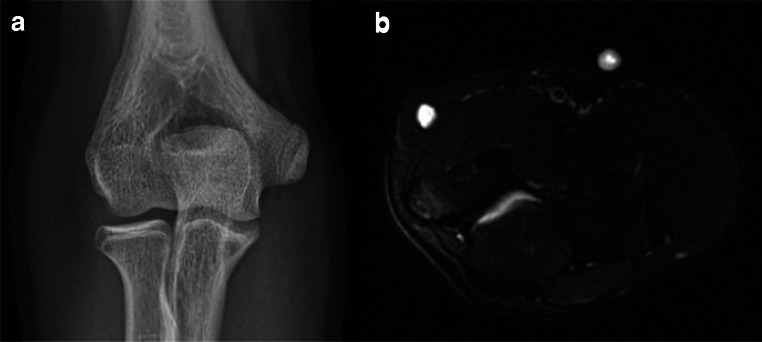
a Anteroposterior radiograph of the elbow in a patient with prolonged pain demonstrates a bulbous medial epicondyle apophysis. b Axial T2 MR image demonstrates bone marrow edema-like signal in the medial epicondyle apophysis and increased signal in the physis with adjacent soft tissue edema. Findings are consistent with medial epicondyle apophysitis
